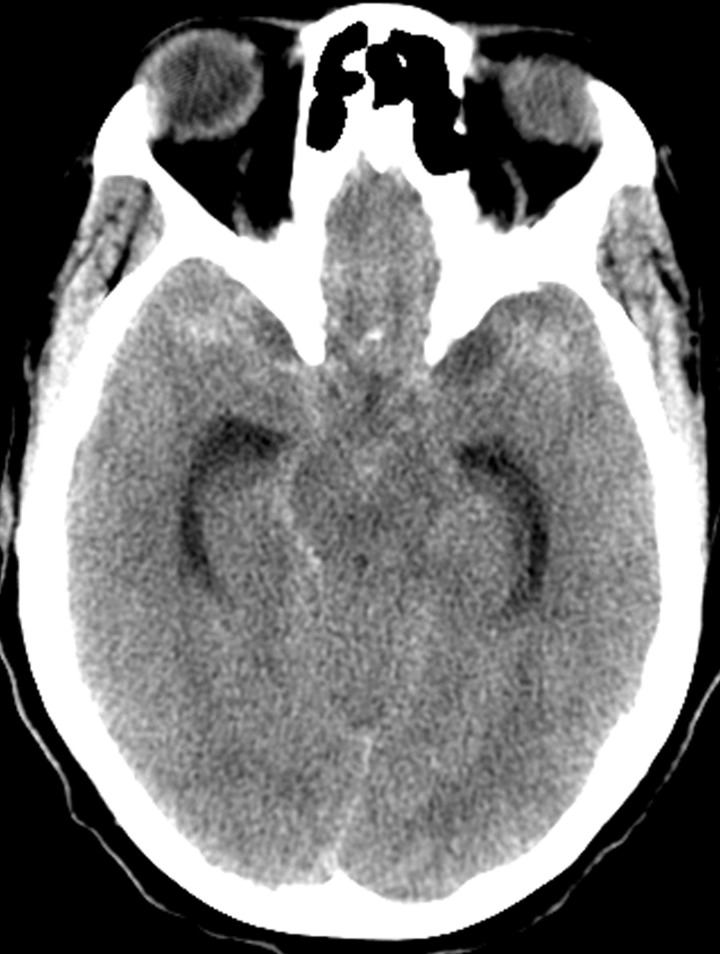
Non-contrast head computed tomography scan demonstrates diffuse bilateral subarachnoid hemorrhage. The volume and distribution of the blood is more than seen in a benign perimesencephalic hemorrhage.
